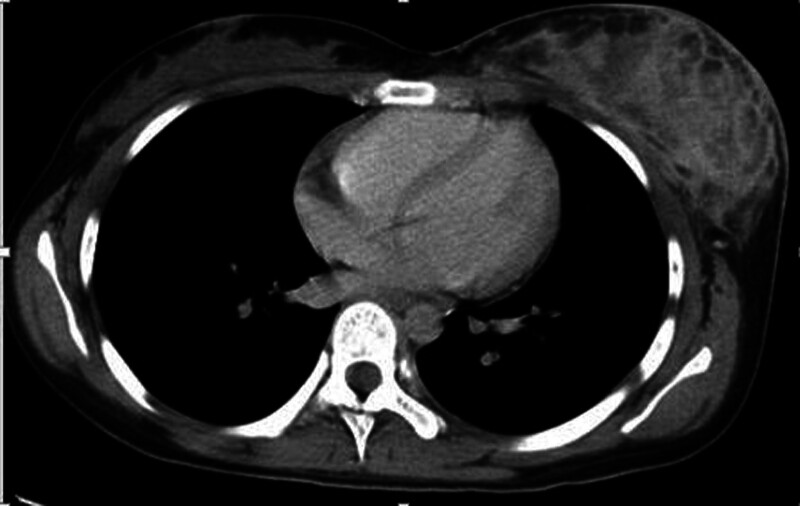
The enhanced computed tomography (CT) scan of the chest revealed multiple cystic abnormal density shadows in the lower outer quadrant of the left breast, exhibiting heterogeneous enhancement and dermal thickening.
